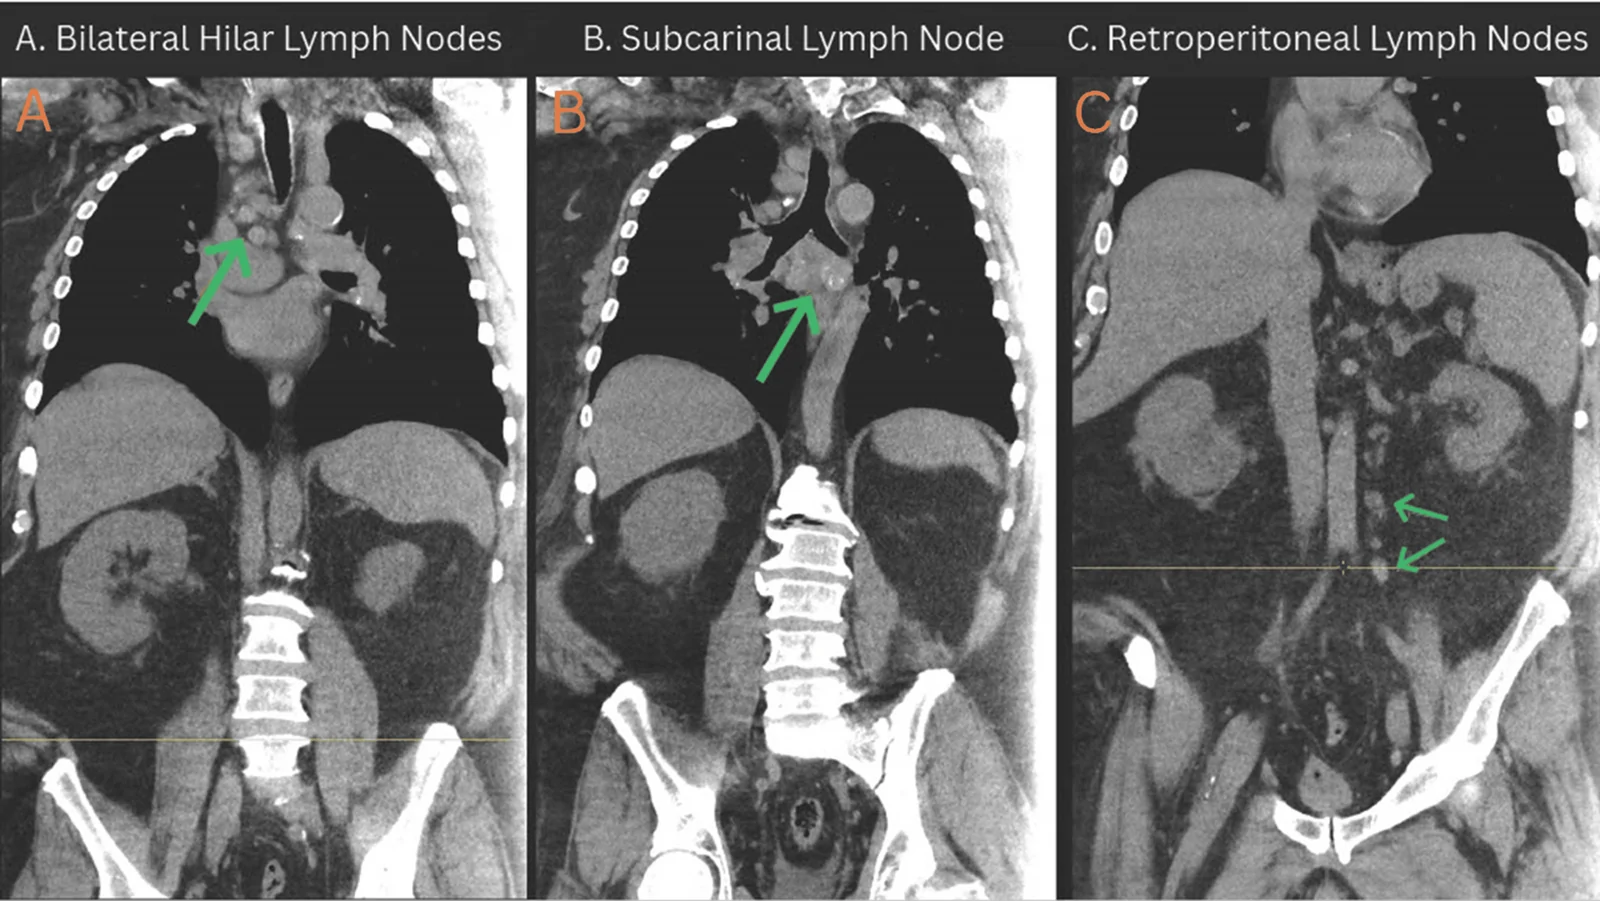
Contrast-enhanced coronal CT images demonstrating lymphadenopathy in multiple nodal stations: (A) bilateral hilar lymphadenopathy (arrow), (B) enlarged subcarinal lymph node (arrow), and (C) retroperitoneal lymphadenopathy in the para-aortic region (arrows) CT: computed tomography
